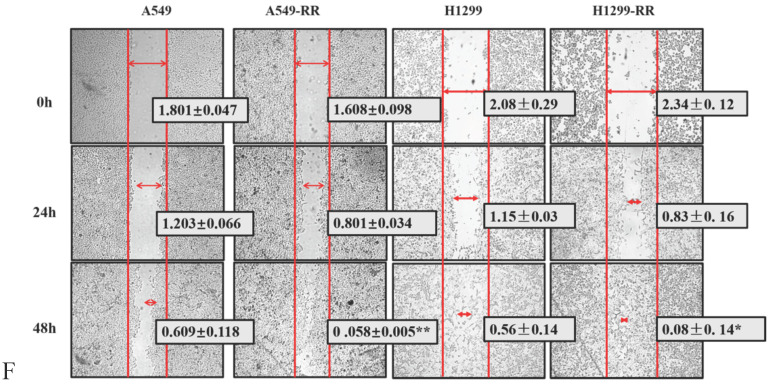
Construction and identification of the radiation resistant lung adenocarcinoma cells. Strategy for the generation of A549-RR and H1299-RR cells (A). Effect of X-ray irradiation on colony forming ability (B) and proliferative ability (C). Morphology changes in A549-RR and H1299-RR cells (D). Changes in cell cycle progression (E). Wound-healing assay of A549-RR and H1299-RR cells (F). A549-RR cells compared with the A549 cells *P* <0.01; H1299-RR cells compared with the H1299 cells ##*P* <0.01 (n = 3).
